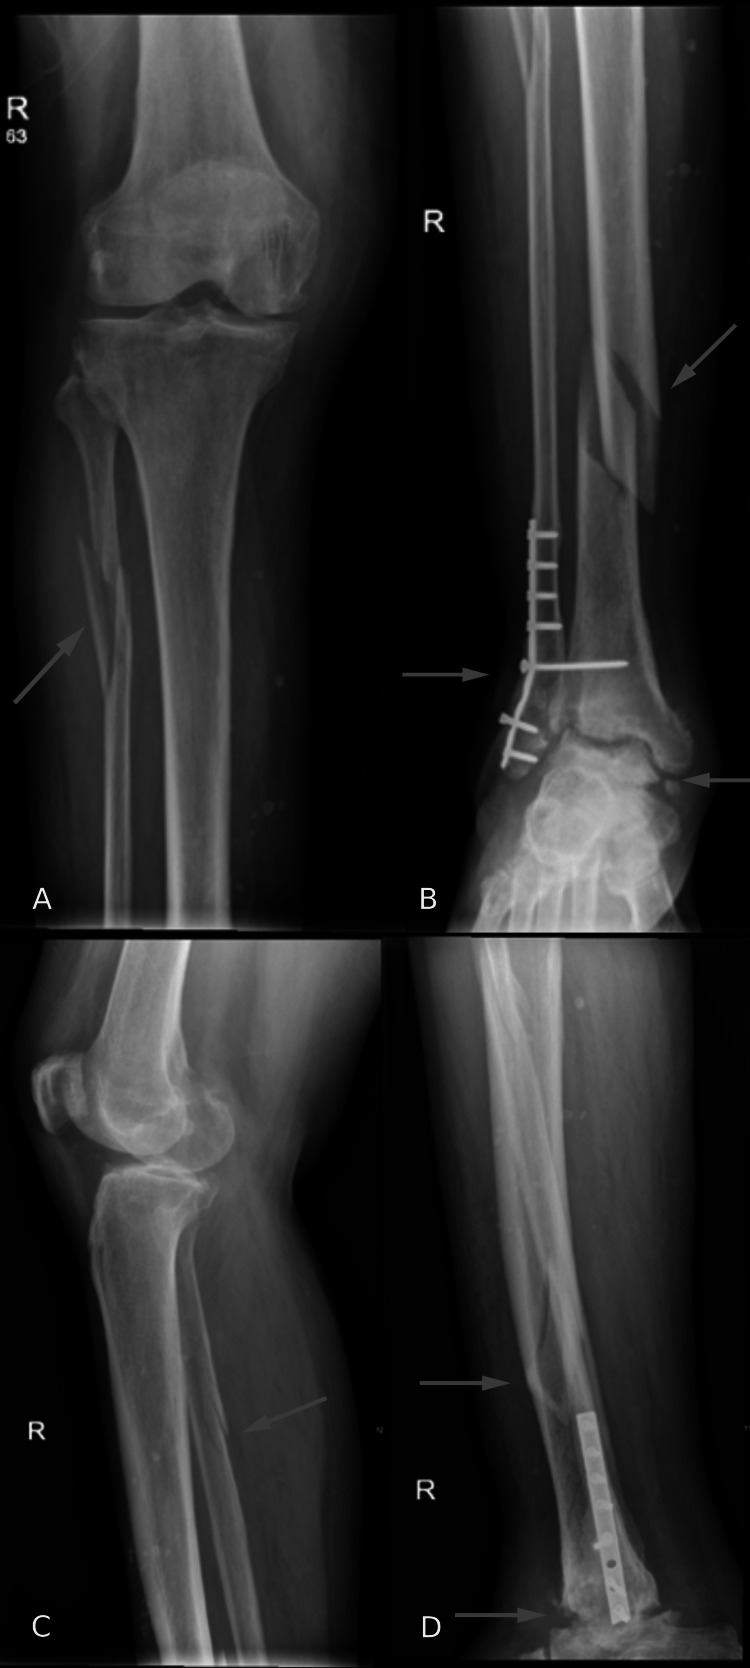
(A, B) Anteroposterior and (C, D) lateral X-rays of the right tibia and fibula after the injury
